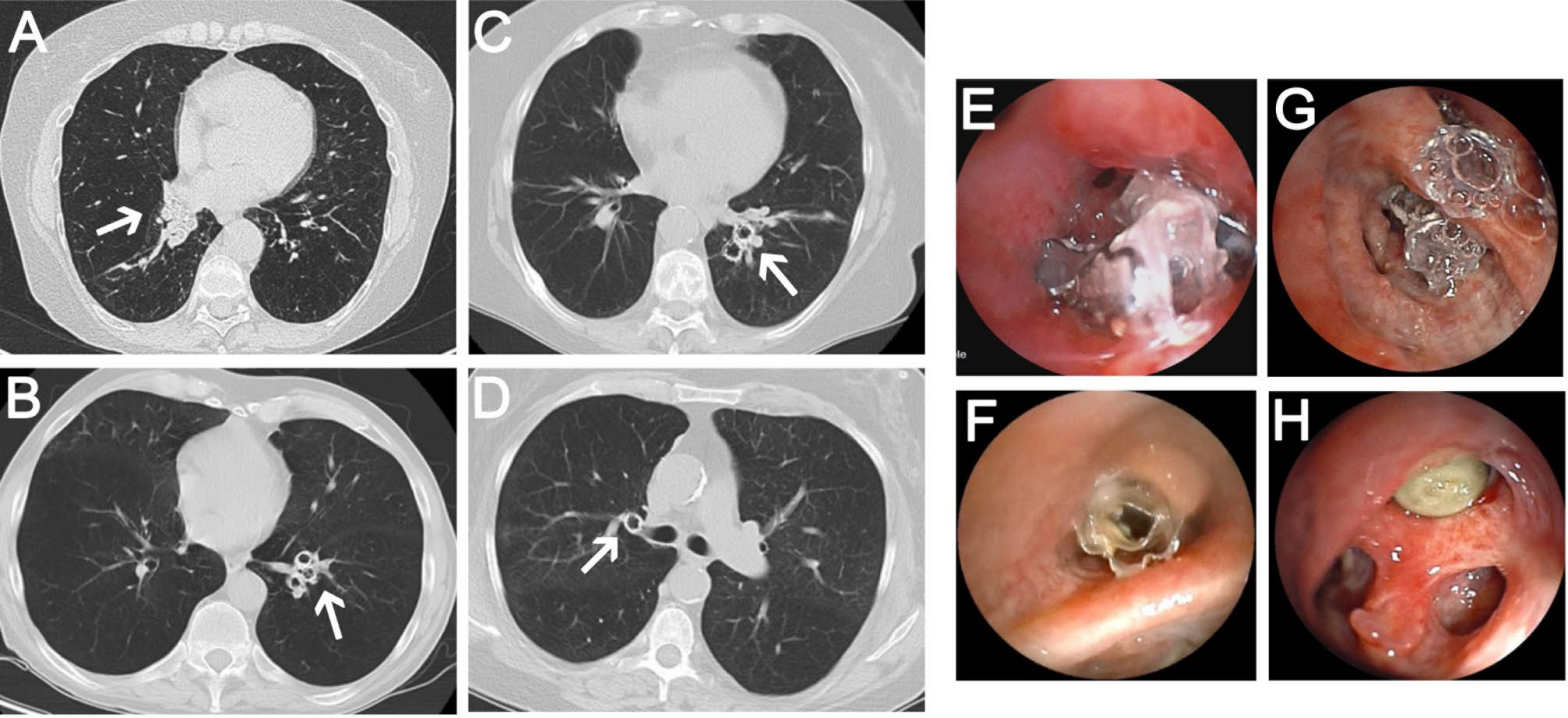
Radiological and bronchoscopic findings in patients after endoscopic lung volume reduction: (A) – CT scans of patient no. 1 with biofilm presented mucus layers on endoscopic valves (EBV) with almost complete obstruction of the right middle lobe. The White arrow points the mucus layer. (B) CT scans of patient no. 2 with biofilm showed signs of mucus layers on EBV in the left lower lobe, indicated by the white arrow. (C) No mucus layers were observed in CT scans of patient no. 5 and patient no. 6 (D), both without bacterial biofilm on EBV. (E-H) Bronchoscopy visualized mucus layers on EBV in patient no. 1, 2, 5 and 6.
